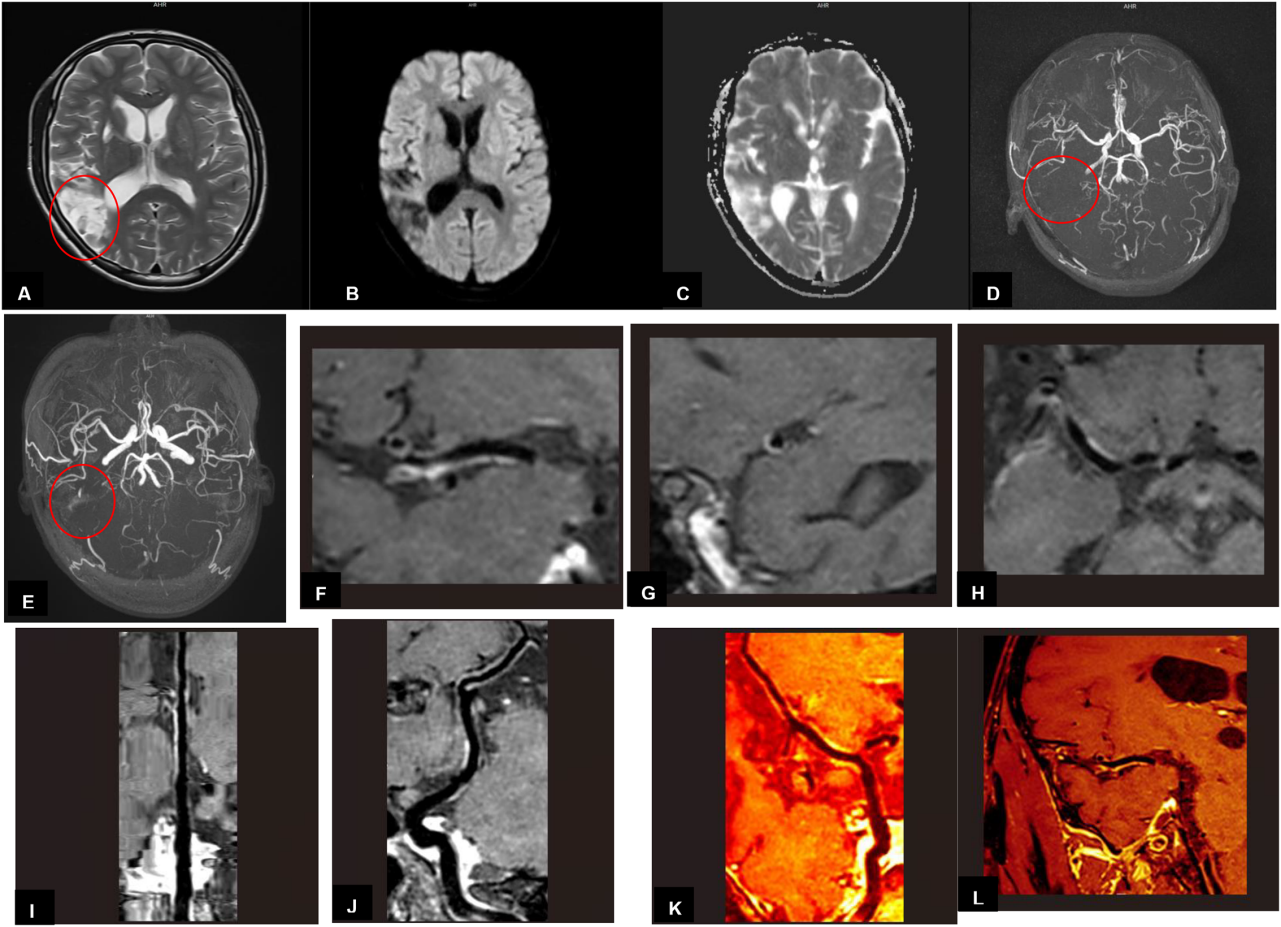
(A-D) Imaging findings obtained 8 weeks after the second discharge. (E-L) Imaging findings obtained 16 weeks after the second discharge (A) MRI findings revealed multiple encephalomalacia foci with high intensities on T2-weighted image in the right temporal and parietal lobes. (B) MRI findings on DWI image in the right temporal and parietal lobes presented no limited diffusion. (C) MRI findings on ADC image in the right temporal and parietal lobes presented high intensities. (D) MRA revealed that the right middle cerebral artery was thinner than that of the left, with fewer branches. (E) MRA showed a thickened vascular shadow beside the M1 bifurcation of the right middle cerebral artery, its distal end and branches were not visualized. (F-L) Magnetic resonance vessel wall imaging showed homogeneous concentric thickening with enhancement in the M1 segment of the right middle cerebral artery.
